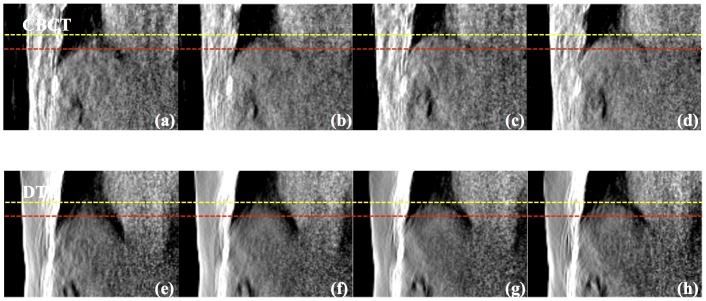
Comparative result (Coronal view) between 4D CBCT and DTS of lung cancer patient #2 case with peak-exhale (a), (e), mid-inhale (b), (f), peak-inhale (c), (g) and mid-exhale (d), (h) phases.
